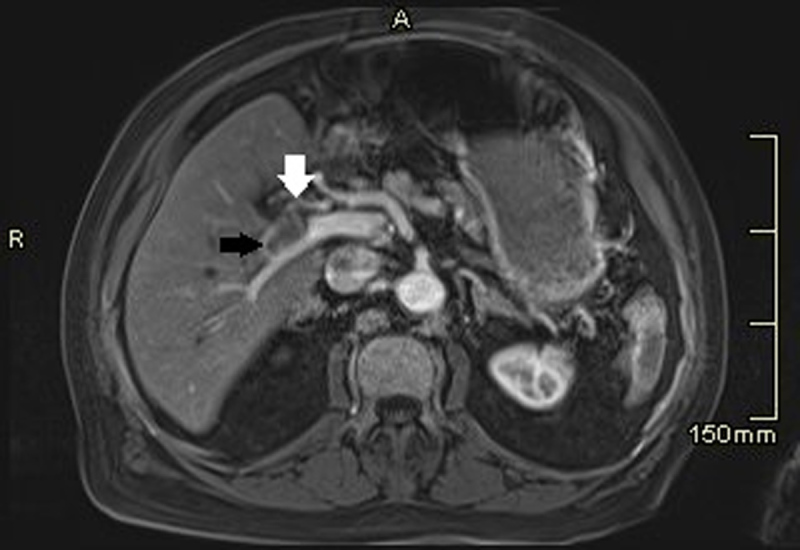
The arterial phase MRI shows this structure (partly visualised; black arrow) deriving from the right hepatic artery (white arrow).
